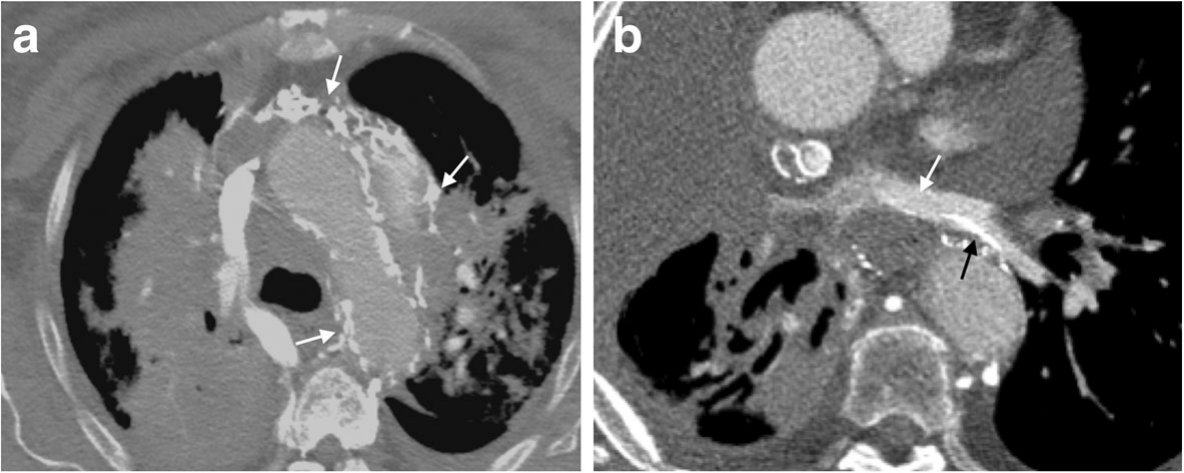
Unusual collaterals. a Axial CT image of a patient with numerous unnamed collateral vessels coursing through the mediastinum (white arrows). b Axial CT scan demonstrates collateral vessels (black arrow) draining into the left pulmonary veins (white arrow)
